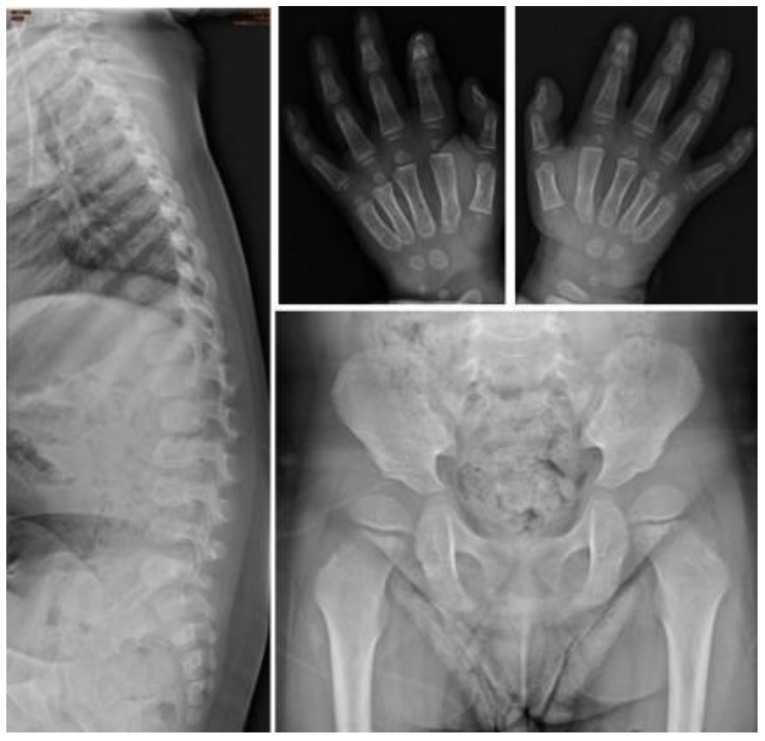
The bone X-rays at 2.8 years of age of patient No. I-6 who had the variation allele c.1007–1666_c.1180 + 2113 delinsTT. ERT was started at 0.4 years of age. He received HSCT at 0.9 years old. At 2.8 years of age, anterior beaking of lower thoracic to lumbar vertebrae, proximal pointed metacarpal and bullet-shaped phalanges of both hands, and shallowed bilateral acetabuli and coxa valga of both femurs were found.
